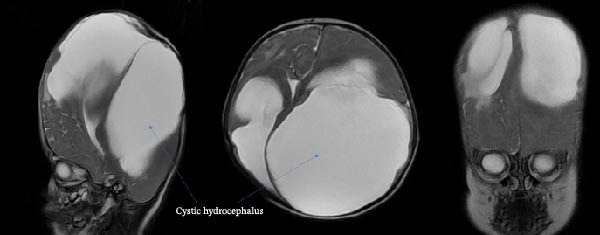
Follow‐up MRI at 6 months postoperatively. 6 months after dural reconstruction and scalp flap repair, the patient developed cystic hydrocephalus.
